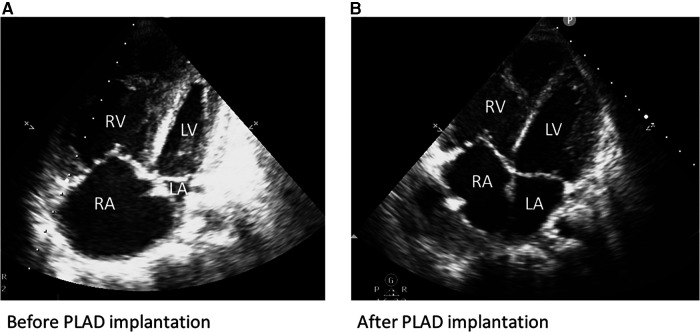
(A) Transthoracic echocardiography before PLAD implantation showing signs of RV failure. (B) Transthoracic echocardiography after PLAD implantation: recovery of RV function and LV volume conditioning.
